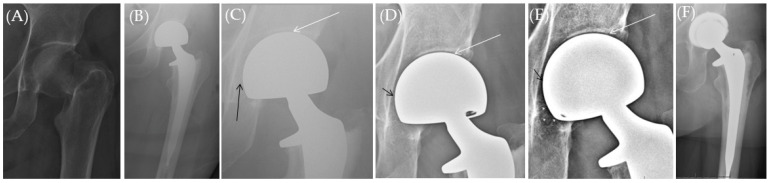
(A) Plain hip X-ray of a 64.7-year-old female (Case 6, Table 4) who sustained a left femoral neck fracture. (B,C) 1-day postoperative plain hip radiograph showing bipolar HA with preserved joint space (white arrow) and preserved medial acetabular bone (black arrow). (D) 6-week, and (E) 18-week follow-up plain radiograph showing stable HA with progressive reduction of the joint space (white arrow), loss of medial acetabular wall (black arrow), and focal bony loss at the acetabular teardrop (asterisks*). The patient eventually received a total hip arthroplasty (F) due to chronic debilitating groin pain.
